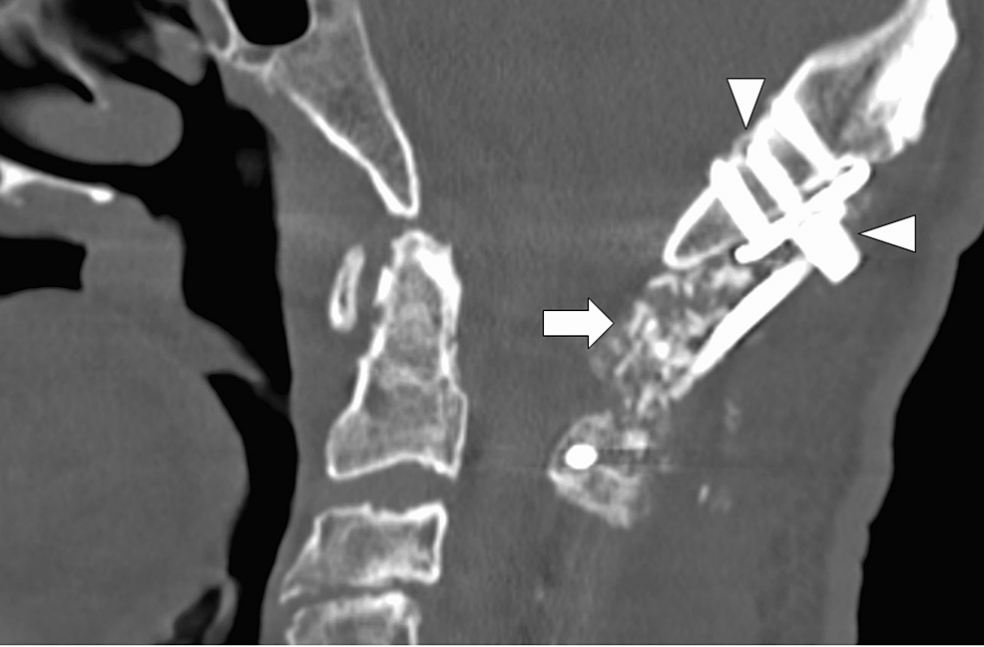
Postoperative computed tomography in case 1 A multiaxial screw is inserted into the contralateral cortex, and the head of the screw is crimped to the plate (arrowheads). Bone grafting is not interfered with by the additional rod (arrow).
